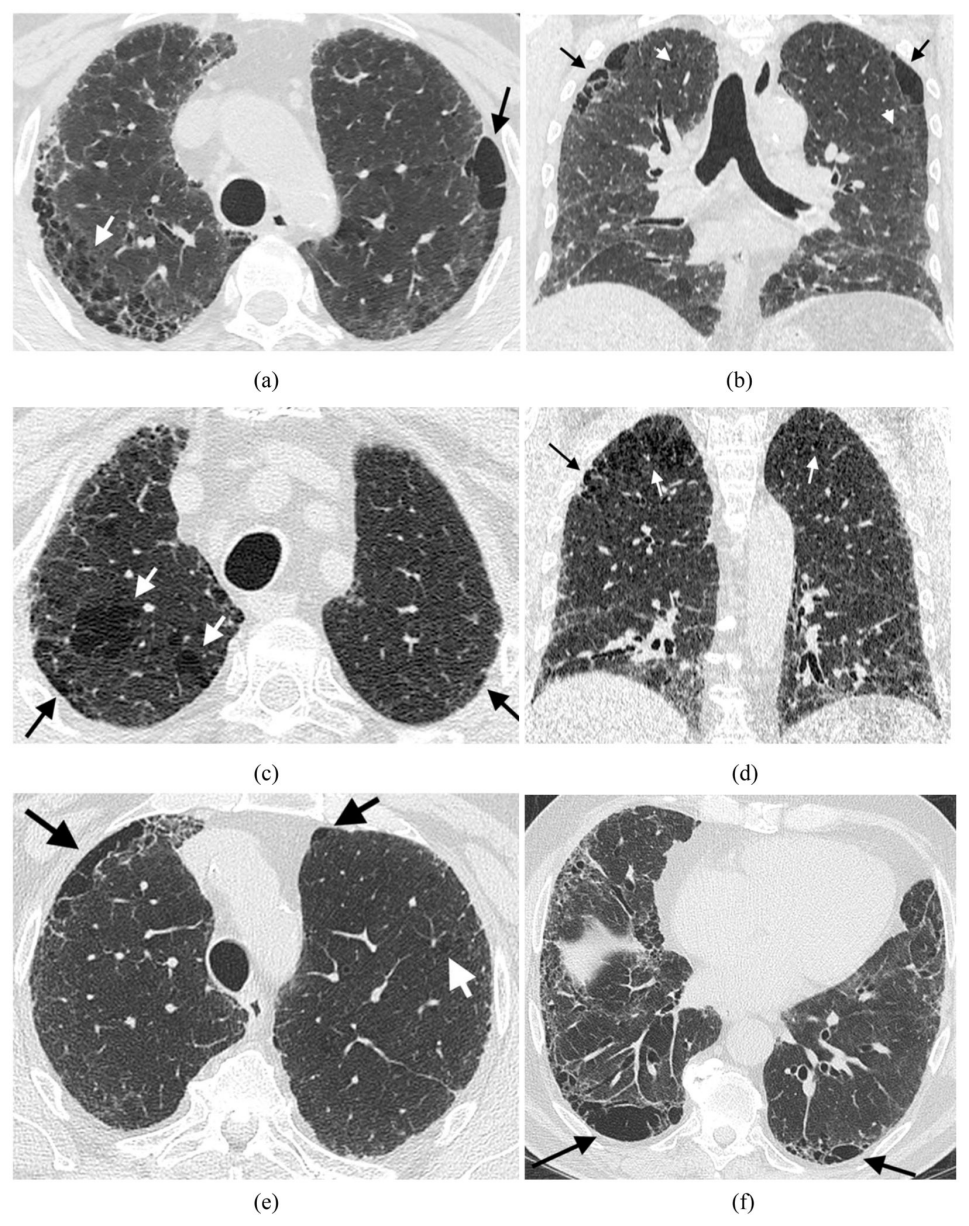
Computed tomography images of three subjects with 10% emphysema scored
